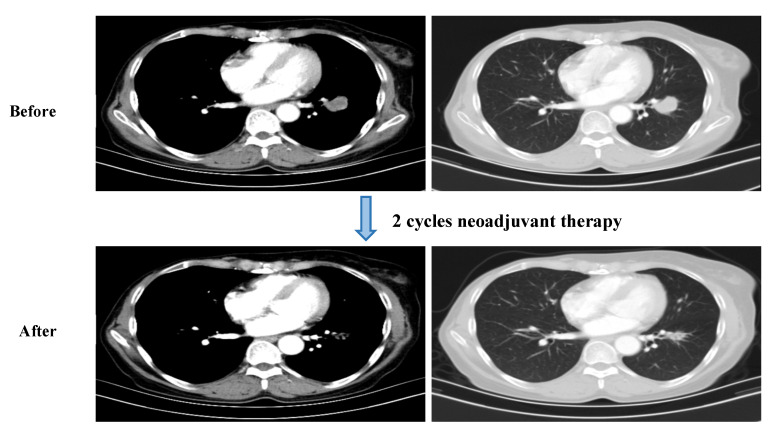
Computed tomography scan of the patient before and after neoadjuvant therapy showed the tumor significantly shrank.
